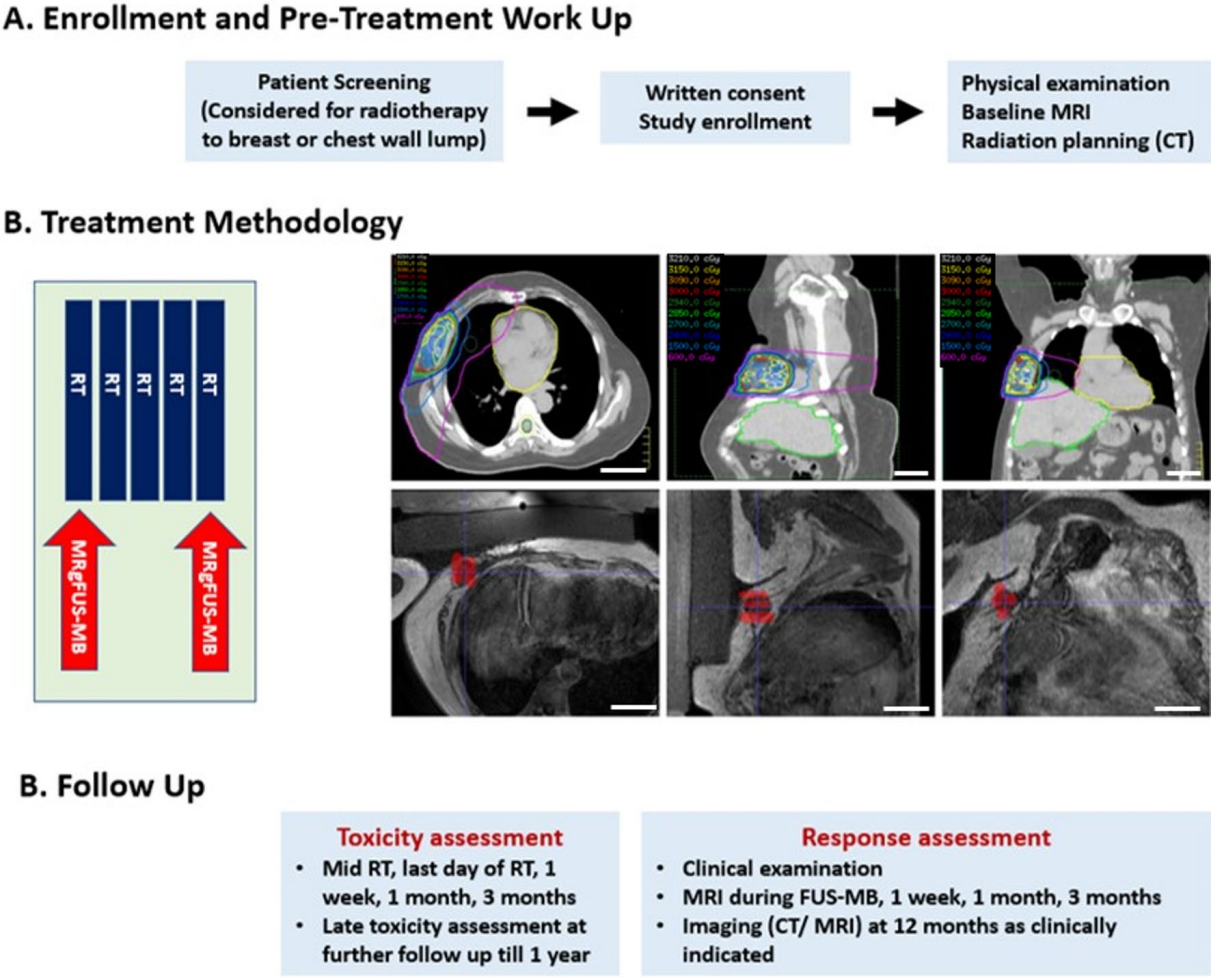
Study methodology, including follow-up and assessment protocols. Section B (methodology) shows the treatment methodology for a patient treated with FUS-MB before the first fraction of radiotherapy. The upper panel of the figures represents the axial, sagittal, and coronal computed tomography images of the radiation plan with radiation isodose lines (as shown in the upper left corner of the individual figures). The lower panel shows corresponding magnetic resonance images with cells (in red) for insonication. The scale on the lower right corner of individual figures represents an approximate size of 5 cm.
